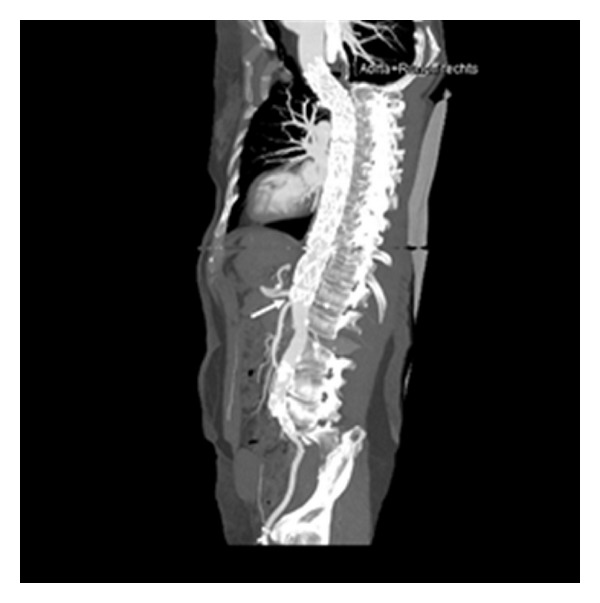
CTA after 3 years and 7 months shows that the distal end of the prosthesis membrane is located immediately proximal to the origin of the celiac trunk (centerline reconstruction, arrow), completely covering the dissection site. The celiac trunk shows physiologic contrast enhancement. No signs of recurrence are visible.
